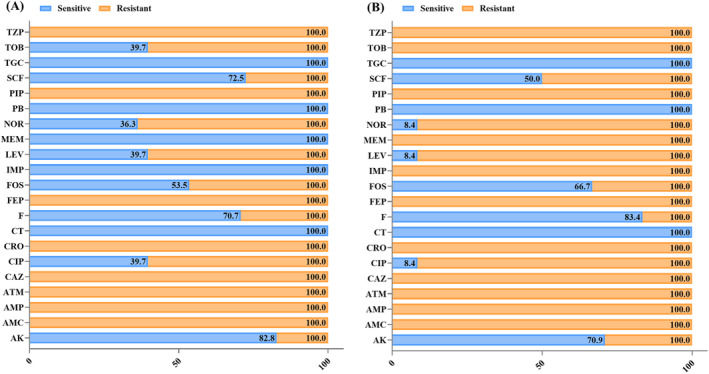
Antibiogram of *Escherichia coli* clinical isolates with color‐coded zones highlighting their antibiotic sensitivity or resistance. (A) The AMR profile of ESBLs; (B) The AMR pattern of carbapenem‐resistant isolates. AK, amikacin; AMC, amoxicillin/clavulanic acid; AMP, ampicillin; ATM, aztreonam; CAZ, ceftazidime; CIP, ciprofloxacin; CRO, ceftriaxone; CT, colistin; F, nitrofurantoin; FEP, cefepime; FOS, fosfomycin; IMP, imipenem; LEV, levofloxacin; MEM, meropenem; NOR, norfloxacin; PB, polymyxin B; PIP, piperacillin; TGC, tigecycline; TOB, tobramycin; TZP, piperacillin/tazobactam.
